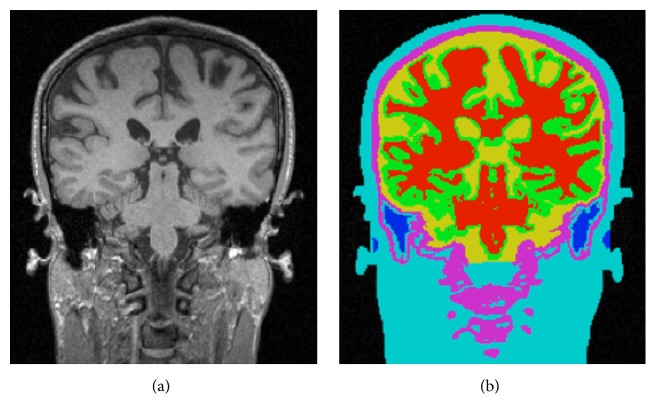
Skull Atlas-to-MRI registration. (a) Transformed MRI. (b) Fusion of Atlas skull and MRI segmentation.
